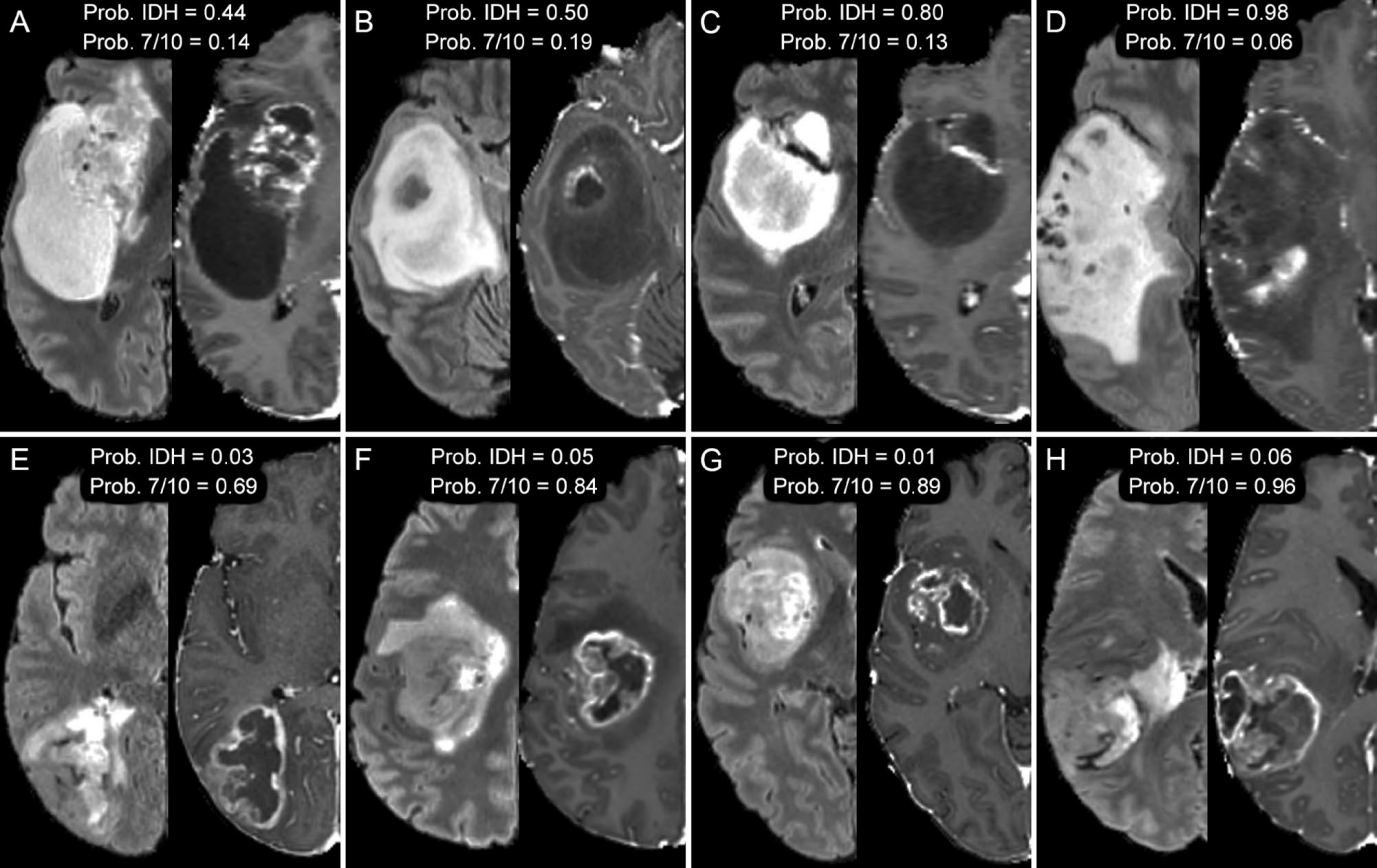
Axial MR images of glioblastomas from 8 different patients, *IDH* mutant (A–D) and chromosome 7/10 aneuploid (E–H). T2-weighted FLAIR images (left) are shown beside corresponding T1-weighted postcontrast images (right). Relative probabilities of *IDH* mutation (Prob. *IDH*) and chromosome 7/10 aneuploidy (Prob. 7/10) generated from random forest regression models are shown for image set of images.
